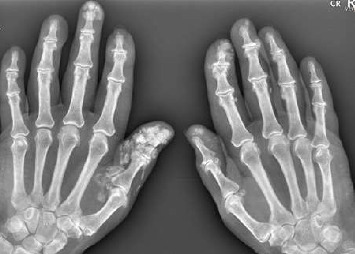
X-ray of the hands: multiple subcutaneous calcifications at the left digiti I and II and the right digitus II. To a lesser extent at right digiti I and III., this can be a part of, for example, dermatomyositis or systemic sclerosis.
